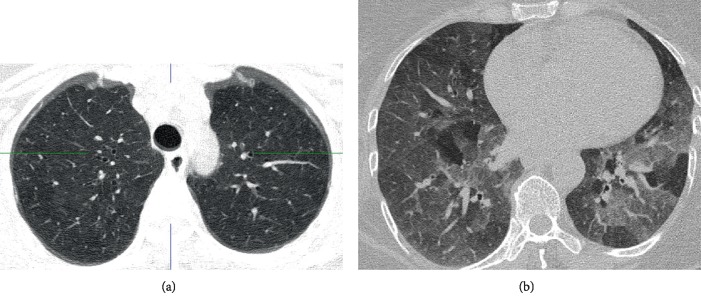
HRCT chest demonstrating upper lobe predominant centrilobular nodules of ground glass density (a). Expiratory HRCT films shows air trapping in lobules that had decreased attenuation on inspiratory film and centrilobular nodules of ground glass density (b).
